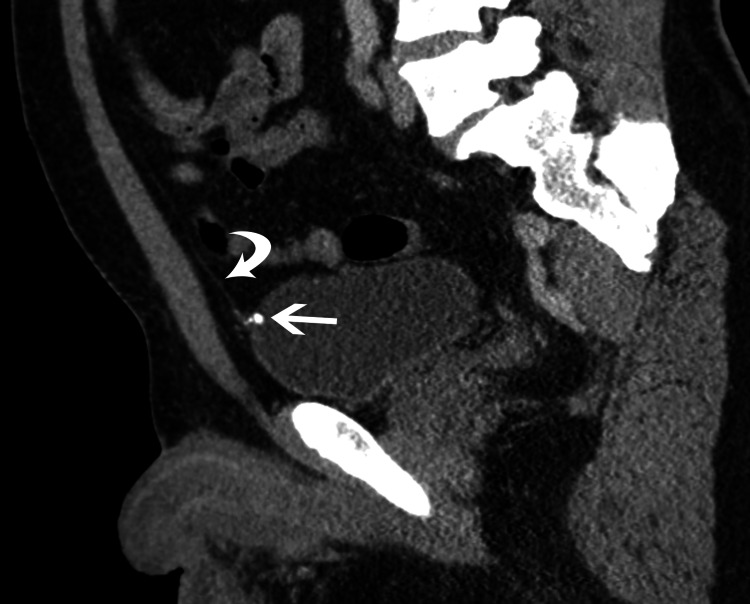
Sagittal reformatted image from CT scan shows cord-like midline structure (curved arrow) extending from the bladder toward the umbilicus representing the median umbilical ligament. The calcifications (straight arrow) are located at the insertion of the ligament onto the urinary bladder. Note that there is no outpouching of the bladder wall at the insertion of the ligament to suggest the formation of a urachal diverticulum.
